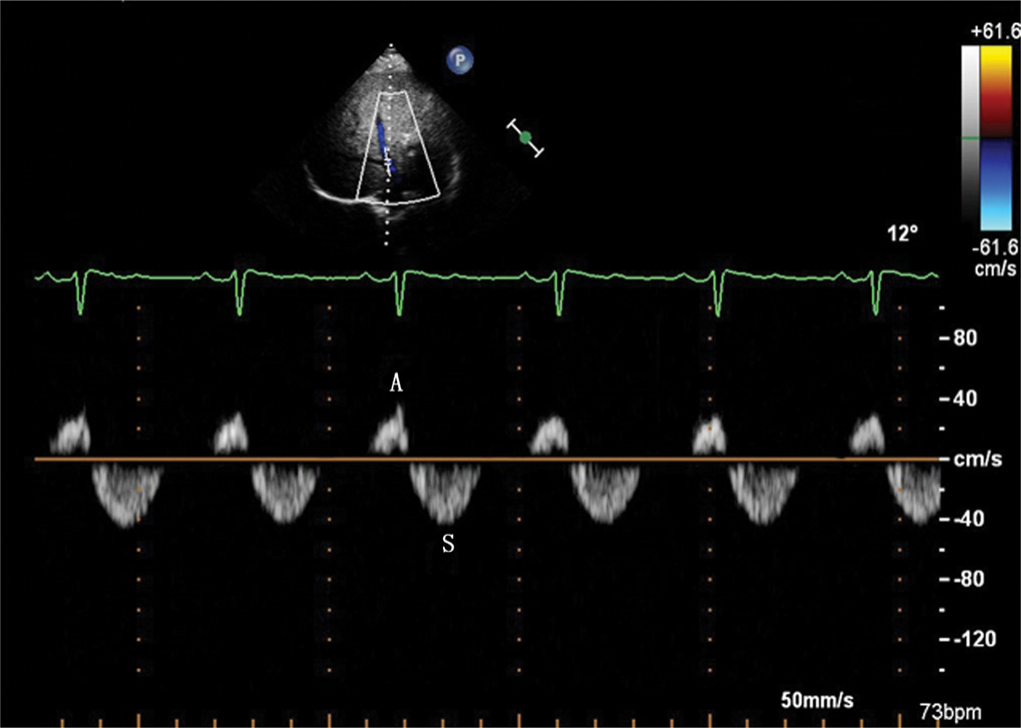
Bi-phasic Doppler flow spectra of the MHV in the PH group. Doppler spectra of MHV obtained from a patient with patent ductus arteriosus with the MPAP of 96 mmHg. The bi-phasic waveform of MHV includes S wave and A wave.
